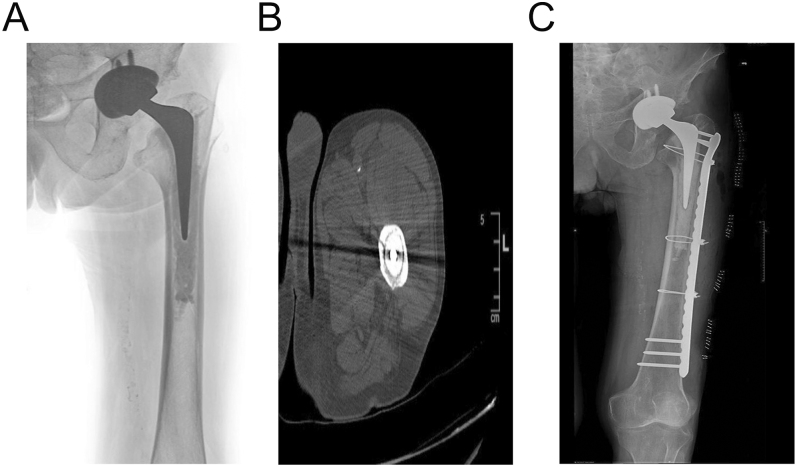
(A) ‘Lucent line sign’ observed in Gruen zone 7, with no other radiographic findings of fracture, in an 86-year-old patient with a low-energy fall and hip pain evaluated at the emergency room. (B) Axial CT scan image confirming a PFF. (C) Postoperative image after ORIF, showing disappearance of the ‘lucent line sign’ at Gruen zone 7.
